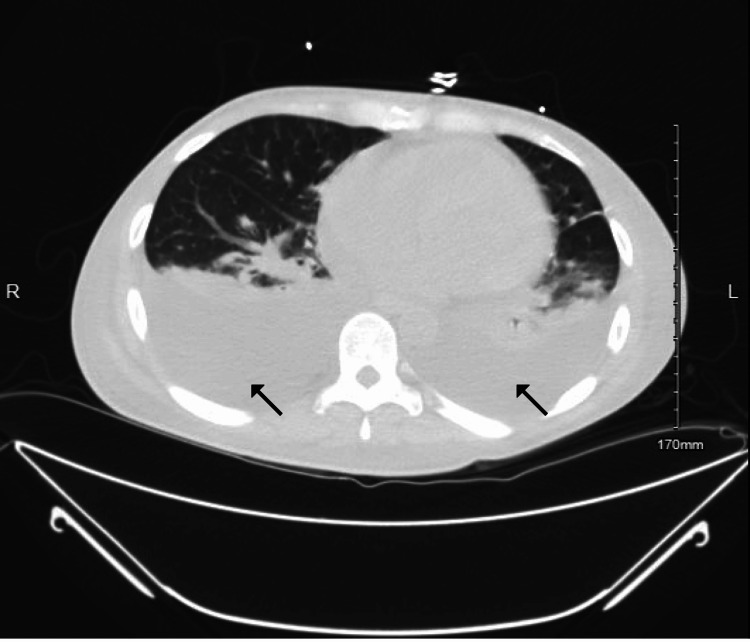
Large bilateral pleural effusions Large bilateral pleural effusions are indicated by the arrows on a non-contrast CT scan of the chest.
